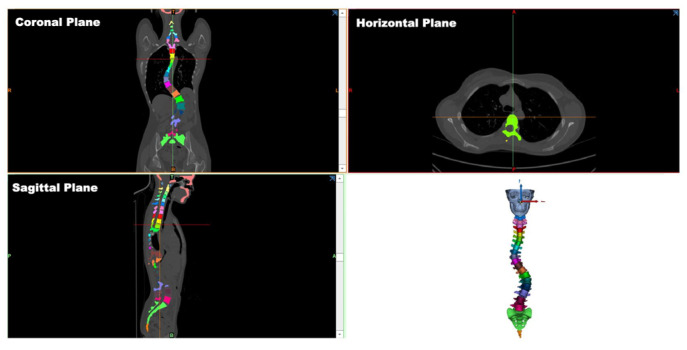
Orthographic views of each segment of the spine on CT images.
